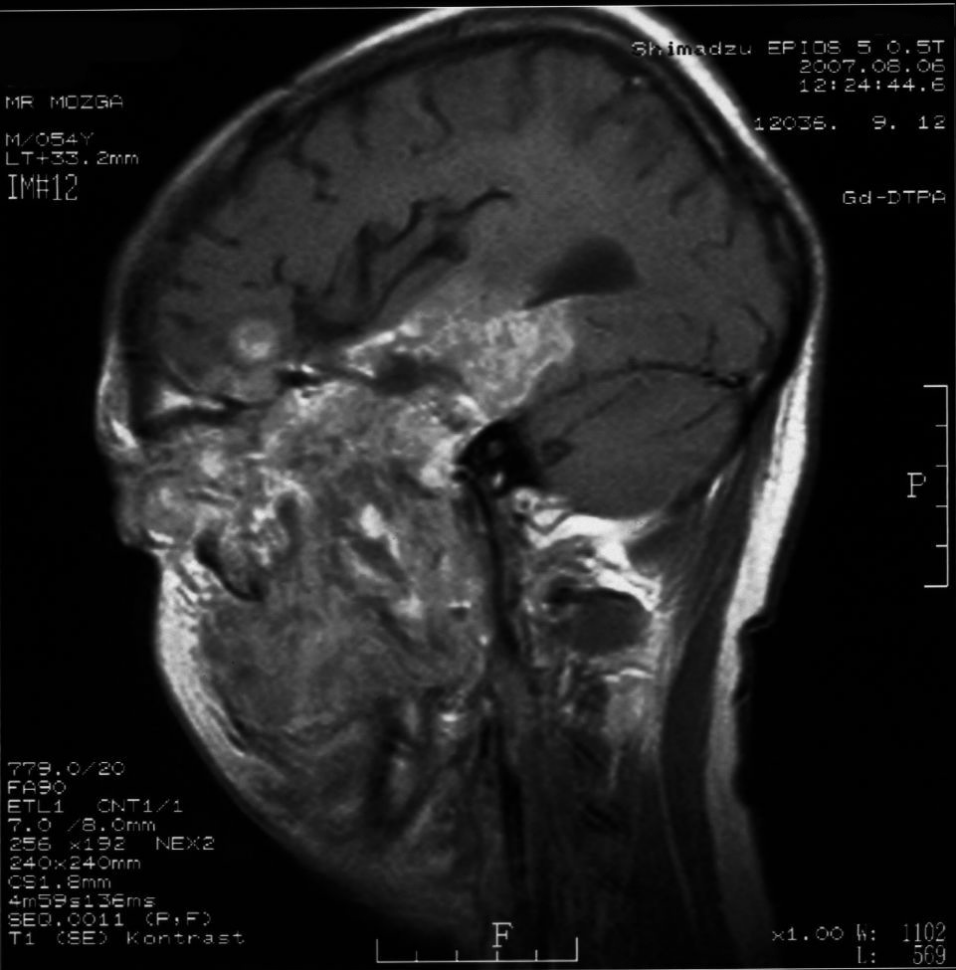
Sagittal T1-weighted contrast enhanced MRI image demonstrates intracranial and extra cranial portion of the tumor extended to the sphenoid and ethmoid sinuses, nasal cavity, orbit, pterygomaxillar fossa, and neck.
